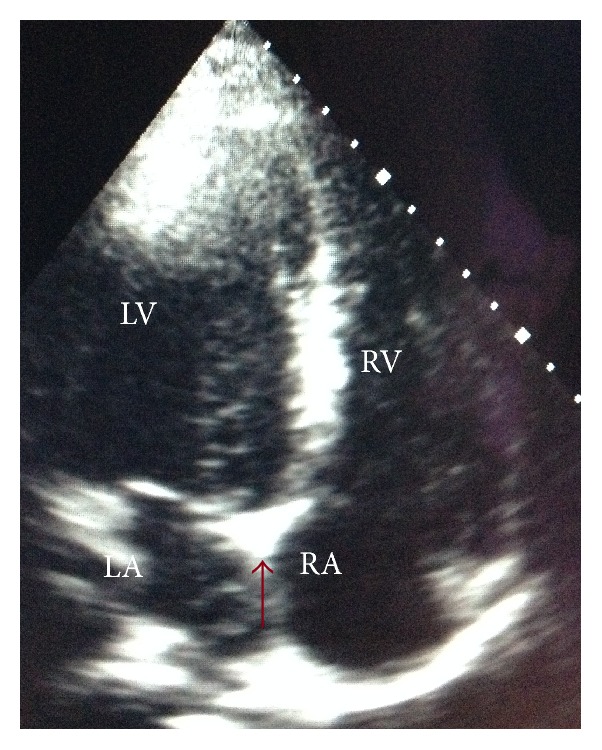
Modified apical 4-chamber view of TTE shows the bullet without pericardial effusion.
